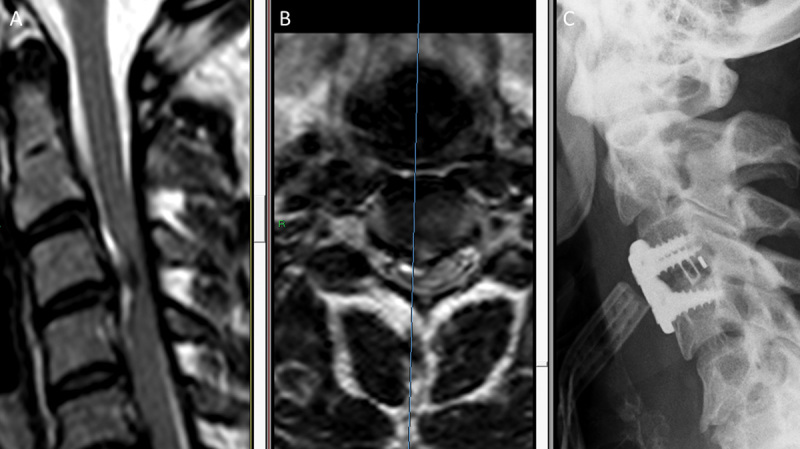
(A) Sagittal and (B) axial T2-weighted magnetic resonance imaging (MRI) scans demonstrate a right-sided acute disk herniation with spinal cord compression and cord deviation to the left in a 32-year-old man. (C) Lateral X-ray performed on postoperative day 1 following C3–C4 diskectomy and fusion with a polyetheretherketone cage and fixed titanium plate.
